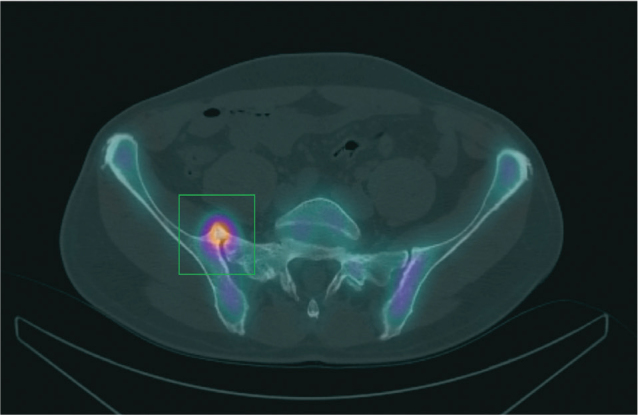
SPECT – CT. This axial SPECT/CT image (fused image of scintigraphy with computed tomography) demonstrates a marked tracer uptake/accumulation at the synostosis at the right sacro-iliac joint (box: tracer accumulation at the synostosis).
